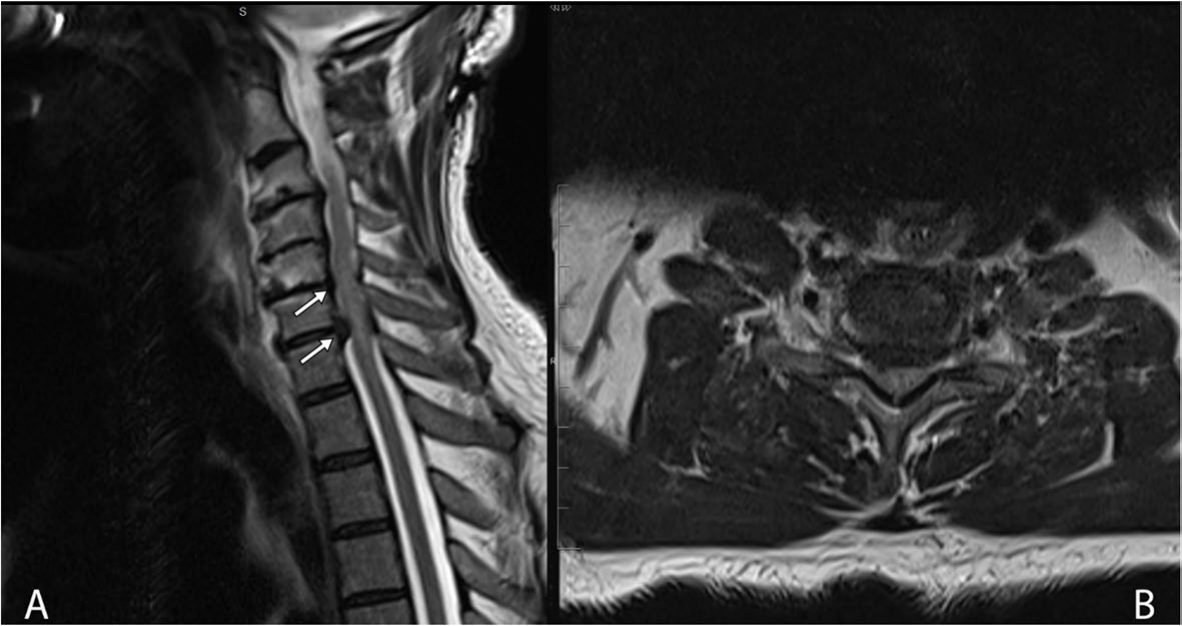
Pre-operative sagittal (A) and axial (B) T2-weighted MRI image of cervical spine demonstrated marked central canal stenosis at the C5/C6 and C6/C7 levels (arrows), resulting in spinal cord compression and myelopathic changes
